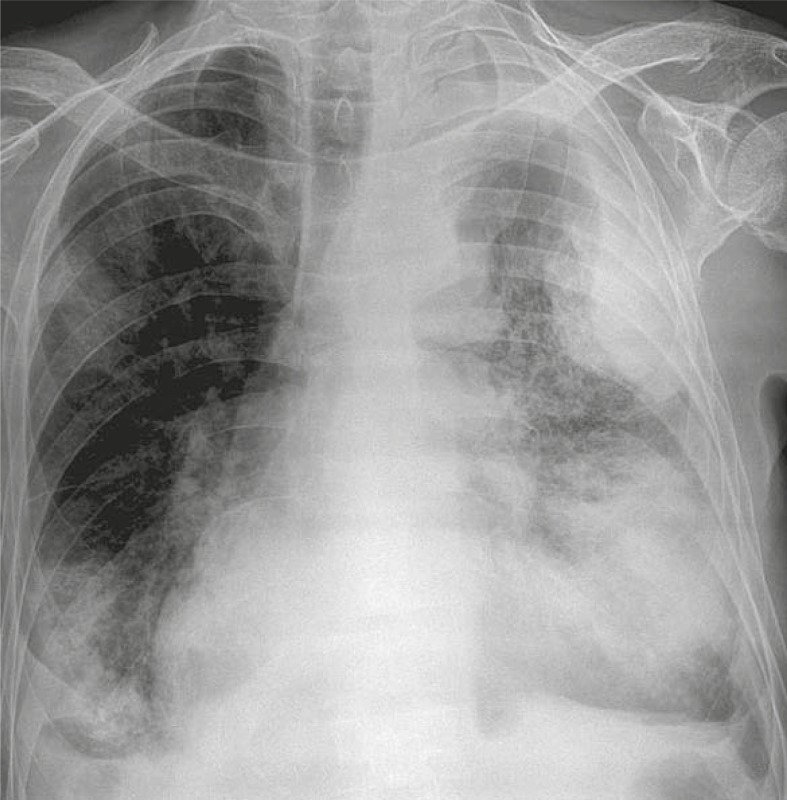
Frontal radiograph shows a bulge projected from anterolateral aspect of the ventricle and pleural effusion.
